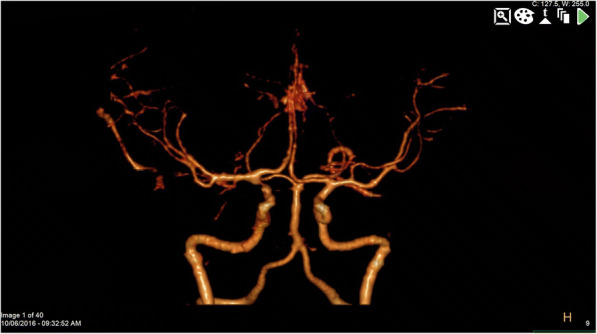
CTA reconstructions at 6 months post-hemorrhage demonstrating persistently occluded aneurysm and resolution of vasospasm
